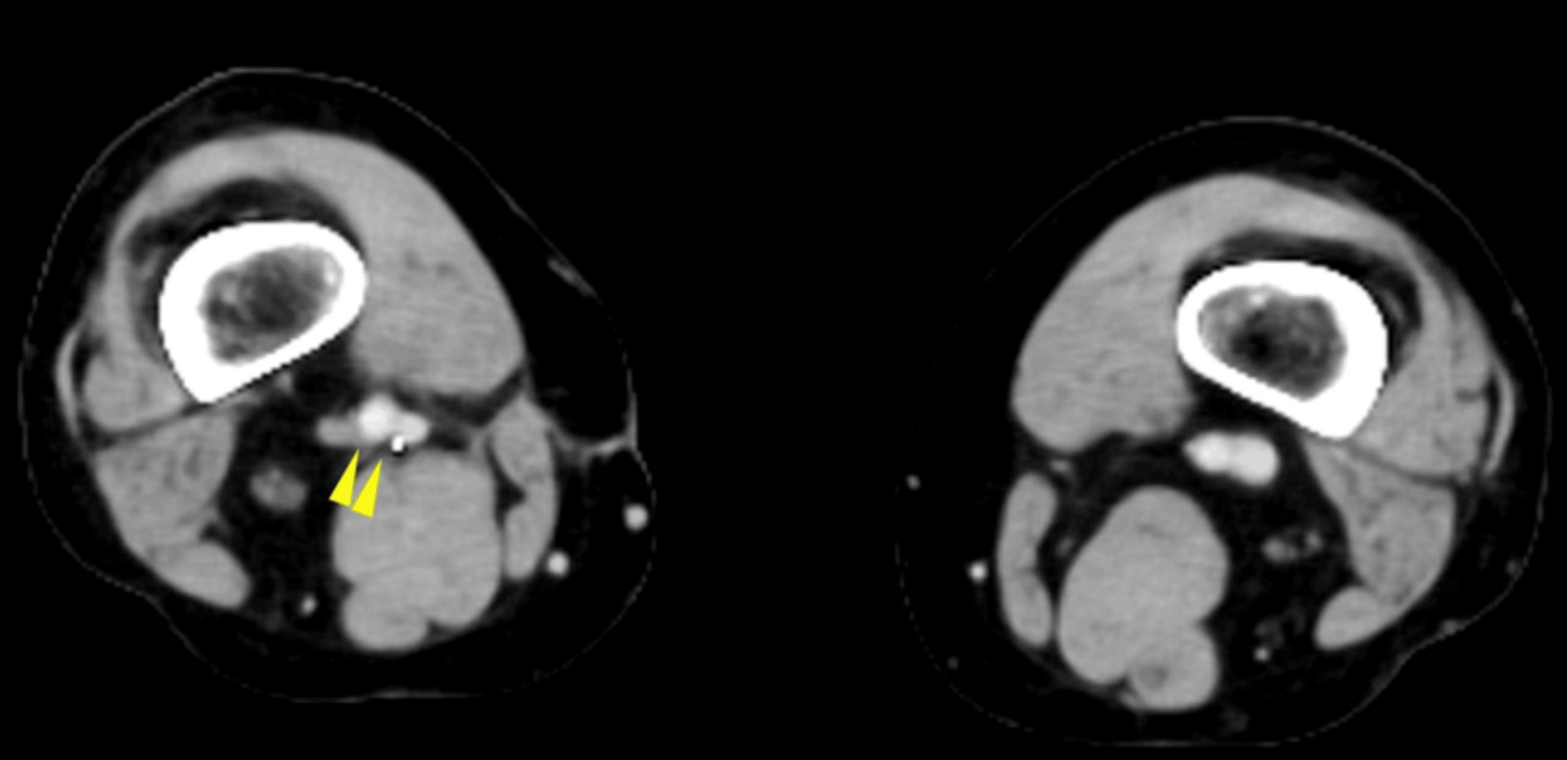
Contrast-enhanced CT at 1.5 years postoperatively. The venous graft remained patent without evidence of stenosis or thrombus (yellow arrowheads). Images were obtained using a 64-slice multidetector CT scanner.
